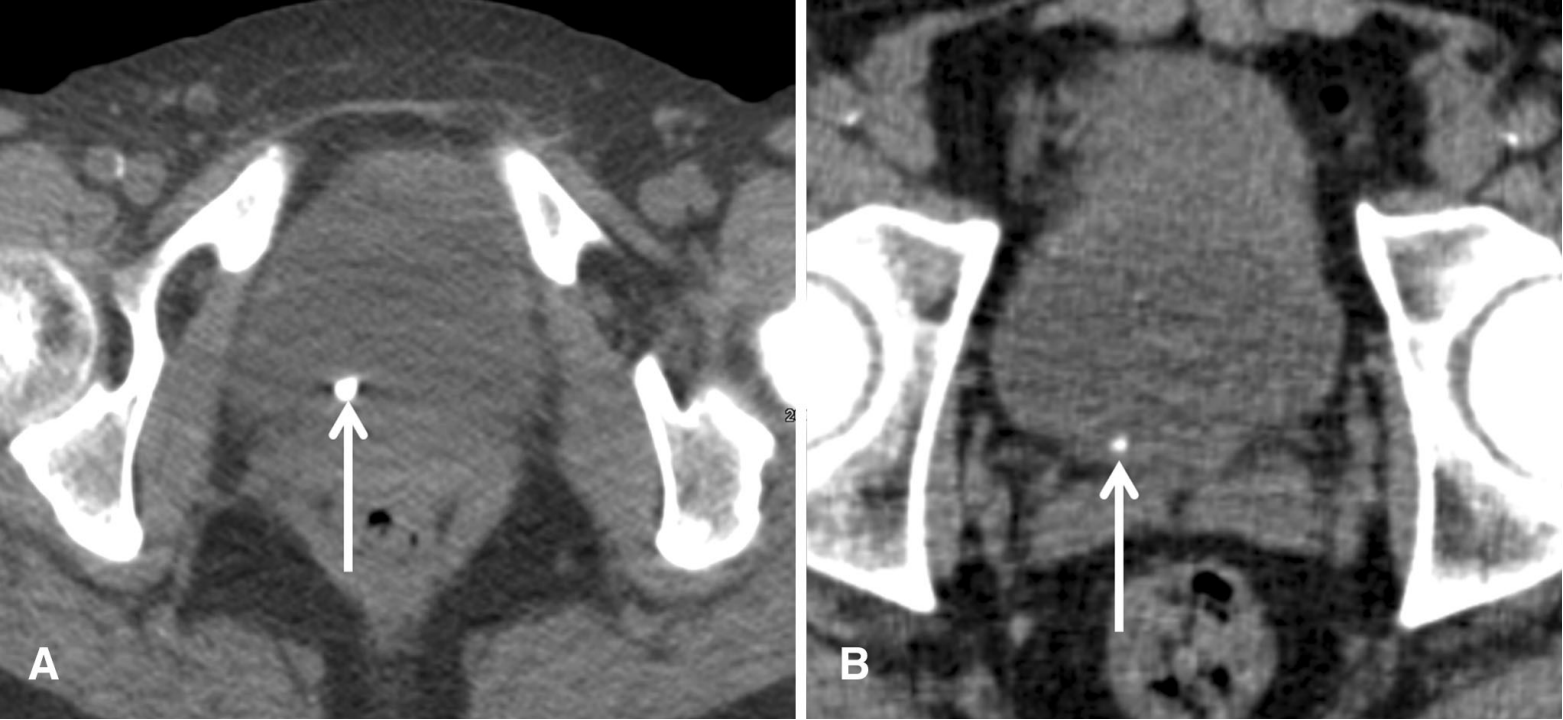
A, B Supine axial unenhanced CT images in two different patients with right-sided acute renal colic and stones in equivocal distal location on CT. On follow-up cystoscopy, the stone in (A) was located in the urinary bladder; the stone in (B) was extracted from the UVJ
